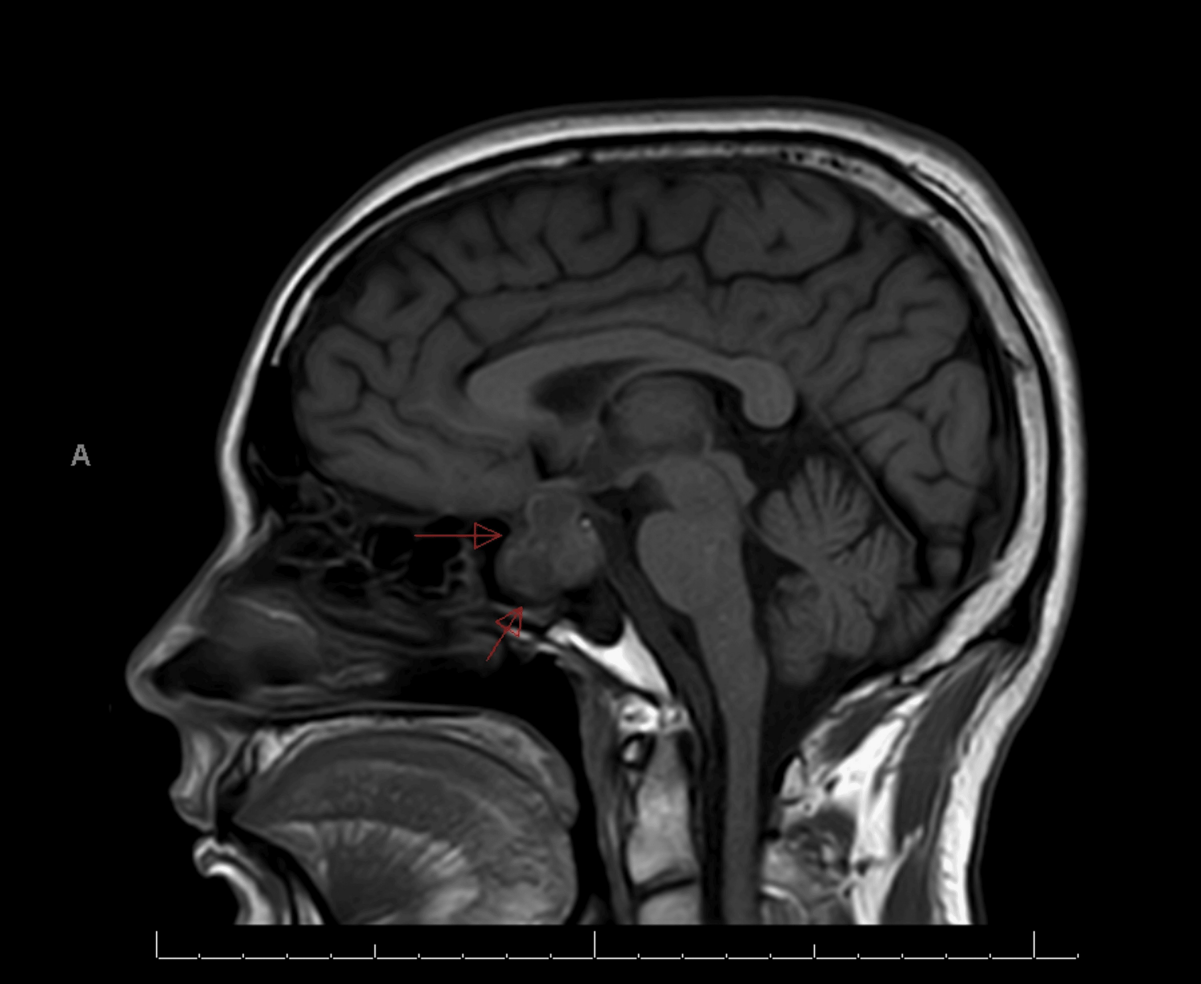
Preoperative magnetic resonance imaging T1-weighted (MRI T1W) sagittal view The red arrows point to the sellar-suprasellar tumor, which has a slightly heterogenous intensity.
